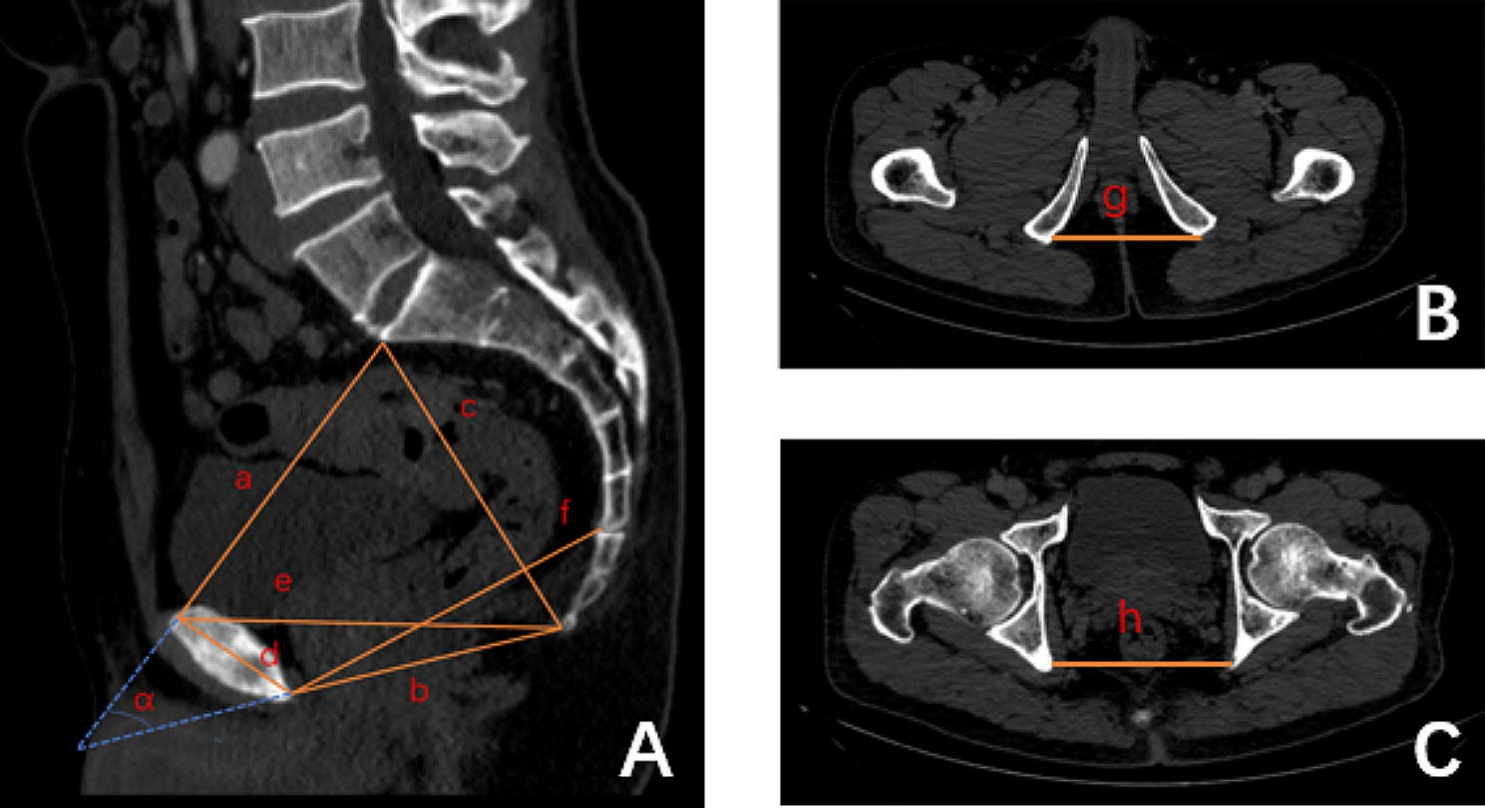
Pelvimetry measured by computed tomography. a: Midsagittal position, anteroposterior diameter of the pelvic inlet; b: anteroposterior diameter of the pelvic outlet; c: sacrococcygeal distance; d: pubic symphysis height; e: distance between the superior margin of the pubic symphysis and the coccyx; f: midpelvic anteroposterior diameter; α angle between the pelvic inlet and extension of the anteroposterior diameter of the pelvic outlet B, C transverse position, g: intertuberous distance; h: interspinous distance
